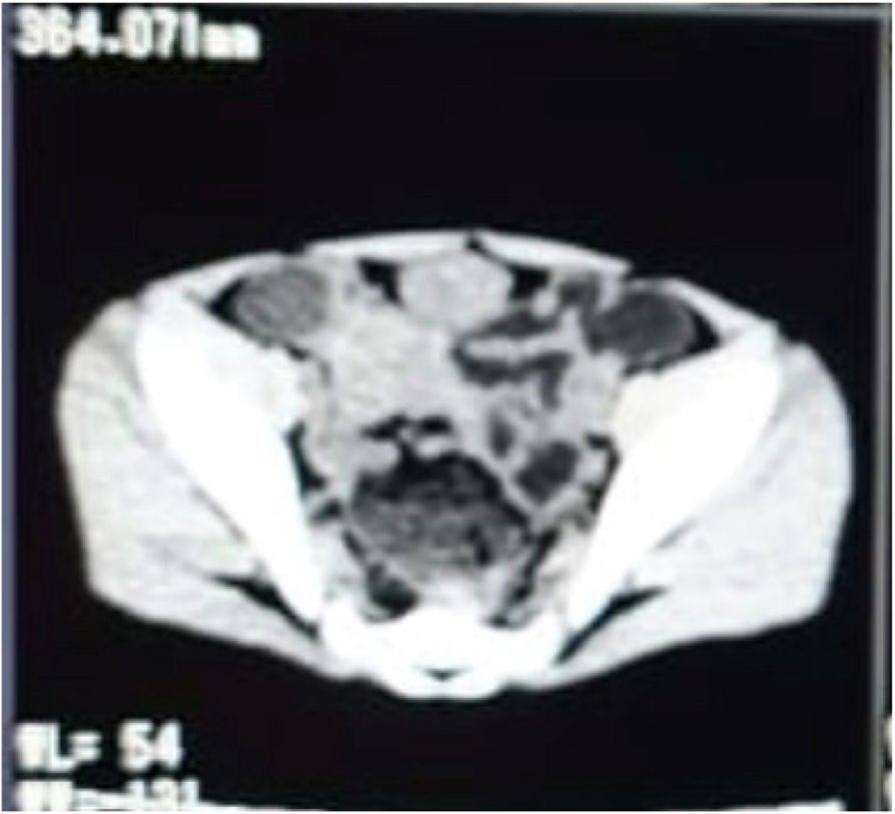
Computed tomography (CT) scan of the abdomen and pelvis showing a thick-walled cyst in the lower abdomen
